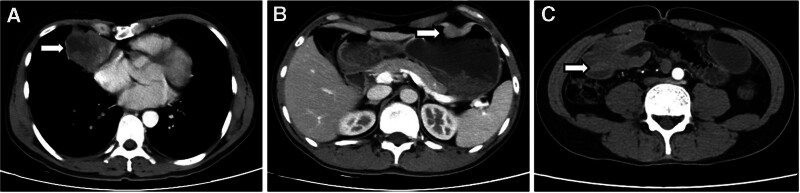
Enhanced CT scan showing a tumor in the right lung (A); malignant tumor on the greater curvature of the stomach (B); intestinal mass and intussusception (C).
